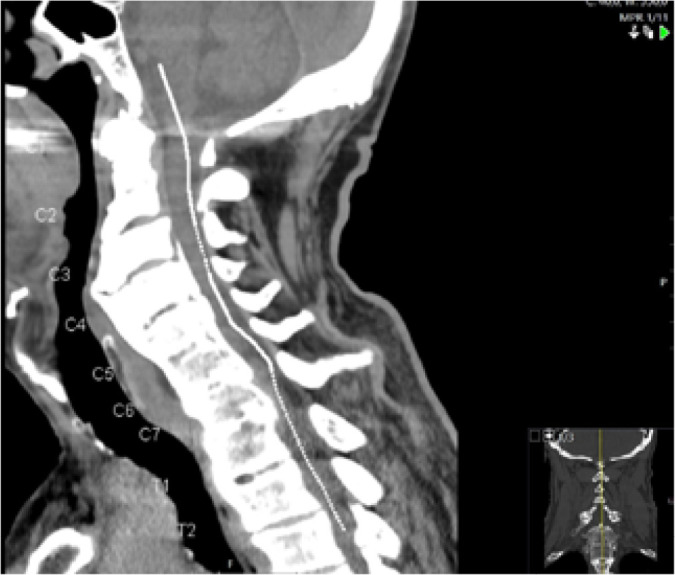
A sagittal Computed Tomographic (CT) scanning cervical spine showing anterior ossification particularly prominent at the level of C3-C4 with indentation on the posterior pharyngeal wall.
